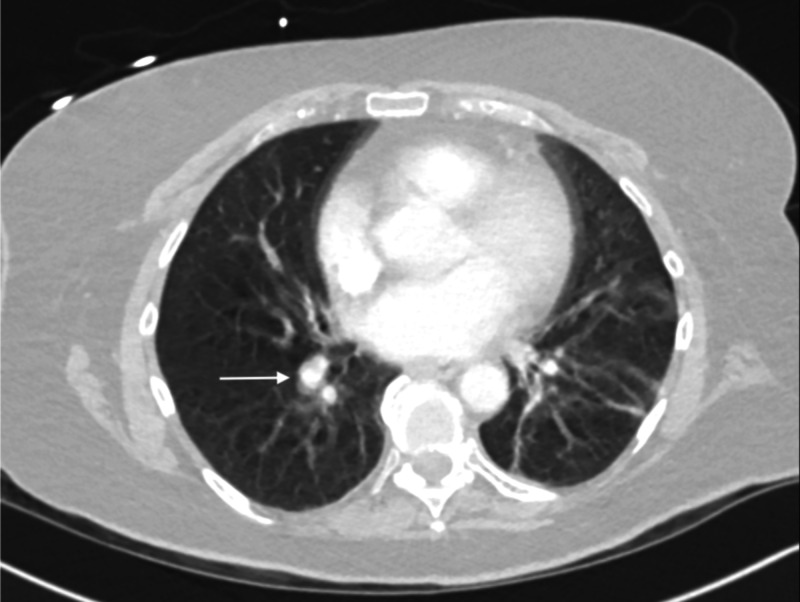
Computed tomography angiogram noting filing defect along the right lower lobe pulmonary artery walls (arrow) consistent with chronic pulmonary embolism appearance
